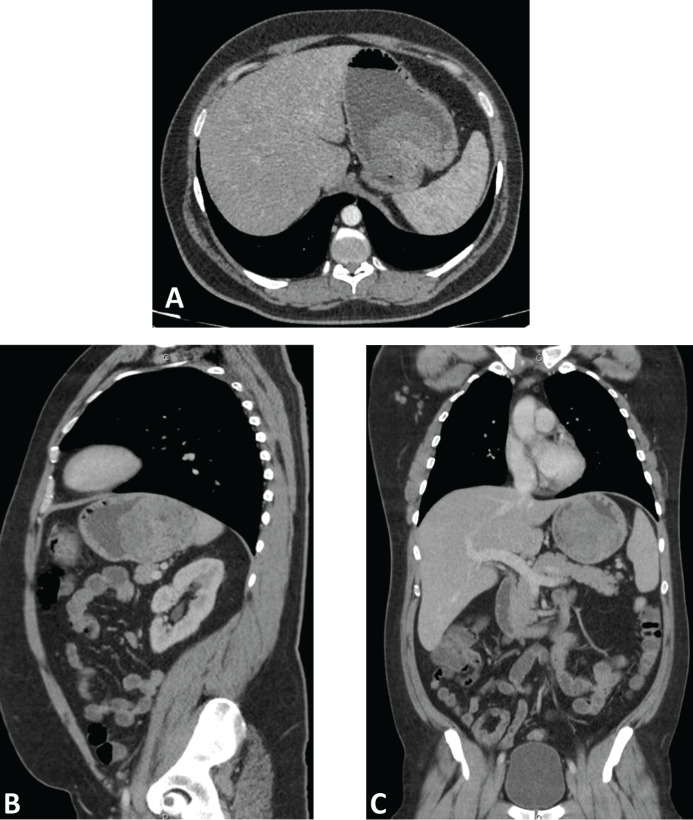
Computed tomography with intravenous and oral contrast. Axial (A), parasagital (B) and coronal (C) planes showing a highly-vascularized tumor with an endophytic growth in the upper gastric body measuring 90 x 89 x 70 mm.
